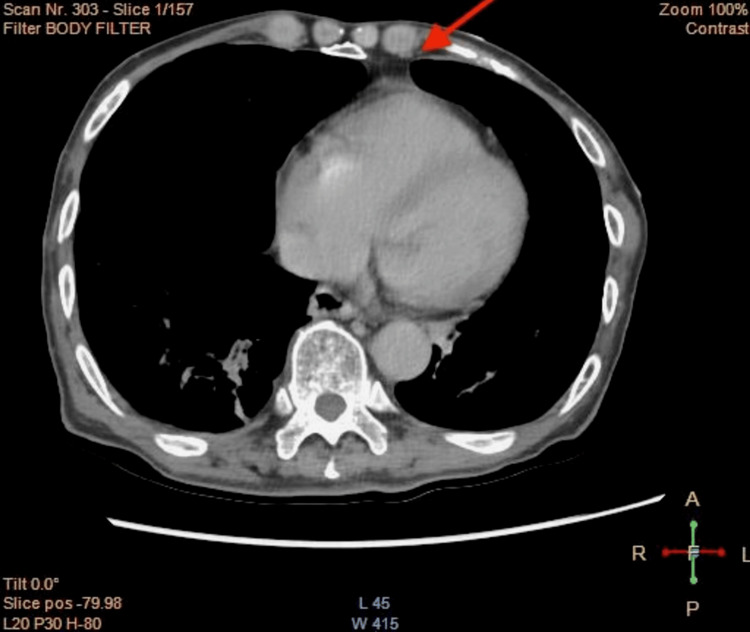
Computed tomography scan with contrast, remarkable for a fluid and fat-containing right inguinal hernia
